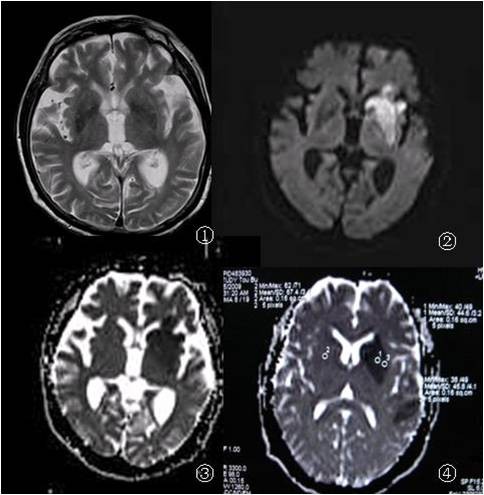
1-3 were taken from one super acute case patient, 4 hours after brain infarction. 1 showed the T2W1 image, which failed to diagnose the infracted region; 2 showed DWI images, with clear infracted region in basal ganglia; 3 is ADC image, showing lower ADC value in infracted region. 4 is acute case 7 hours after infarction, with lower ADC value in the center of infracted region.
